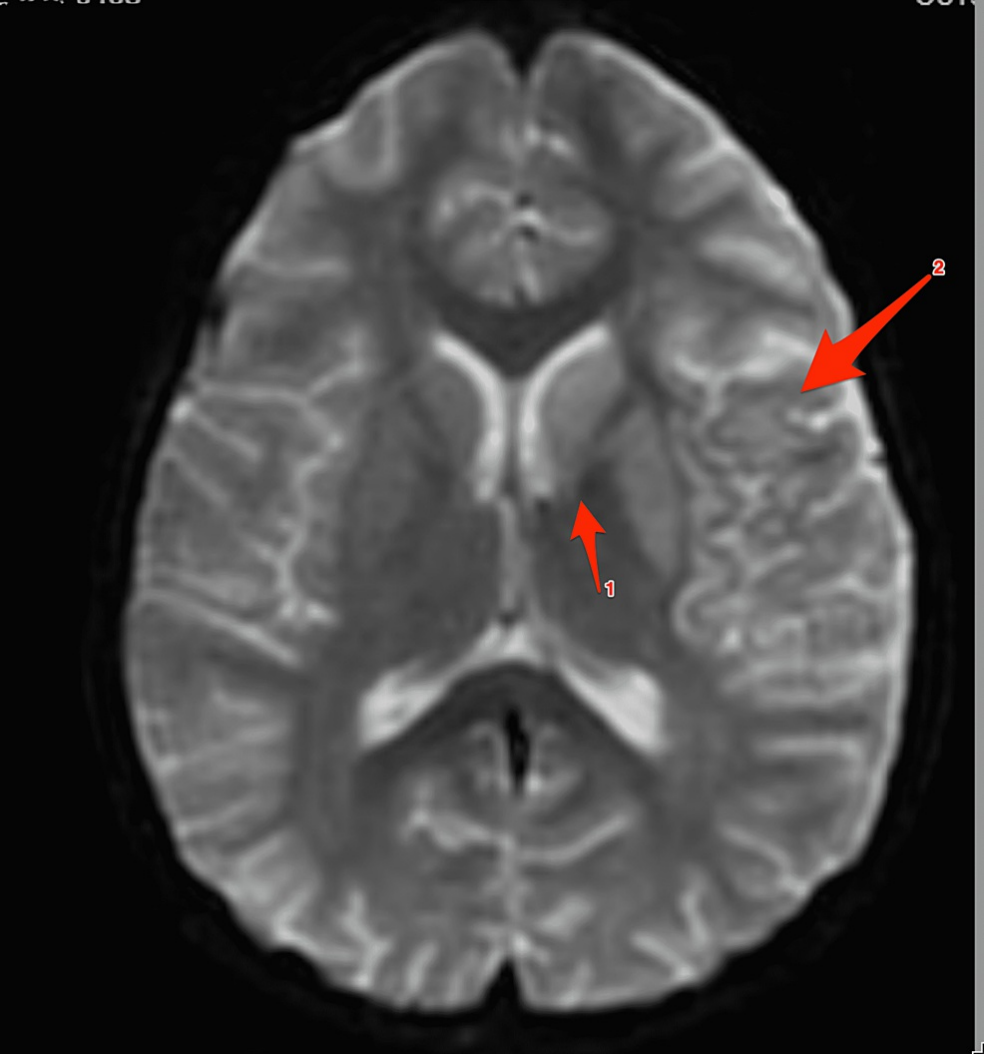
MRI of the brain obtained upon presentation to an outside facility Arrow 1: diffusion restriction at the left caudate nucleus. Arrow 2: diffusion restriction in the left parietal and temporal lobes.
